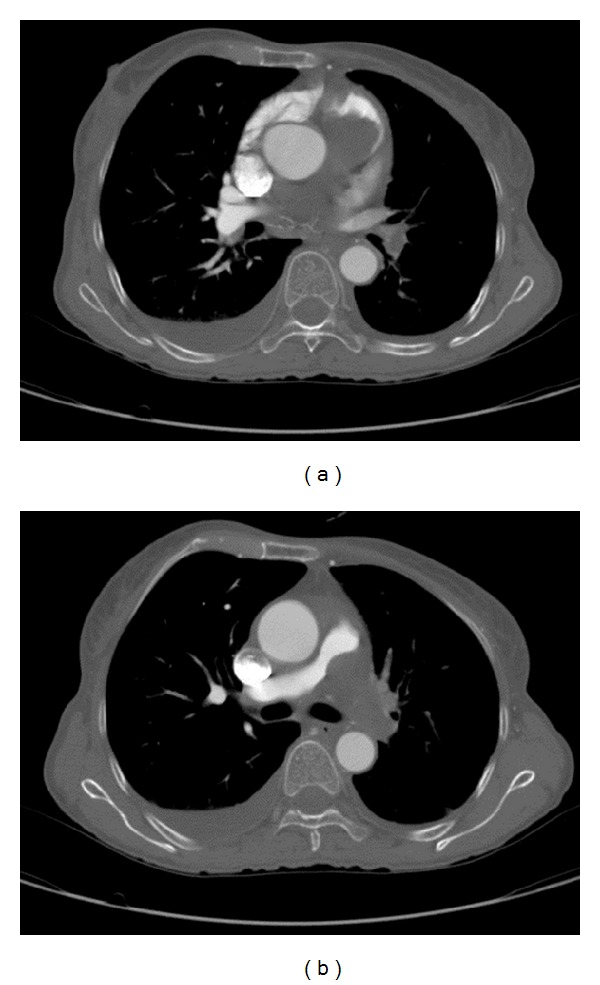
CT scan showing lobulated heterogeneous left hilar mass.
